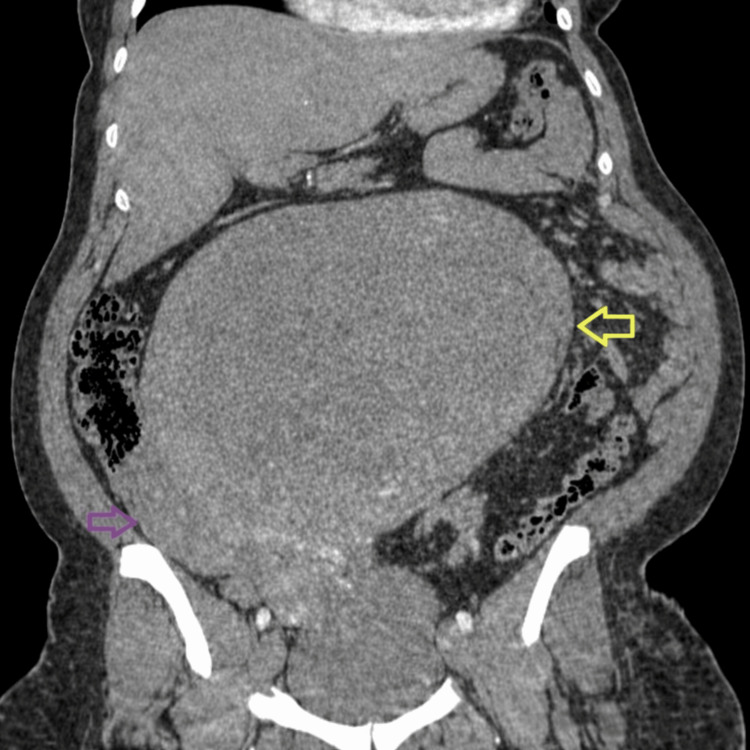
Contrast-enhanced coronal CT of the abdomen demonstrates a large uterine leiomyoma (yellow arrow) and an adjacent cystic lesion of the right ovary (purple arrow).
